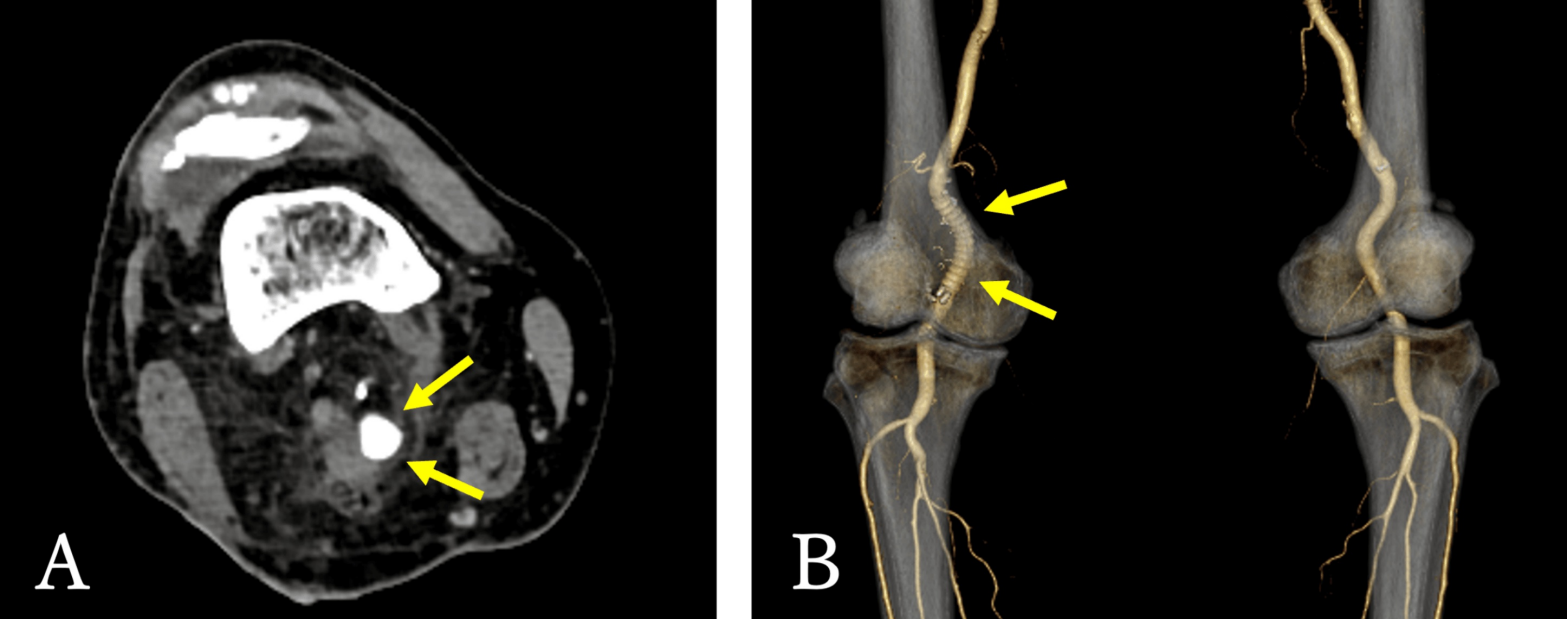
Postoperative contrast-enhanced computed tomography. (A) The right popliteal artery was replaced with an expanded polytetrafluoroethylene (ePTFE) graft (arrows). (B) Improved blood flow in the right lower limb (arrows).
